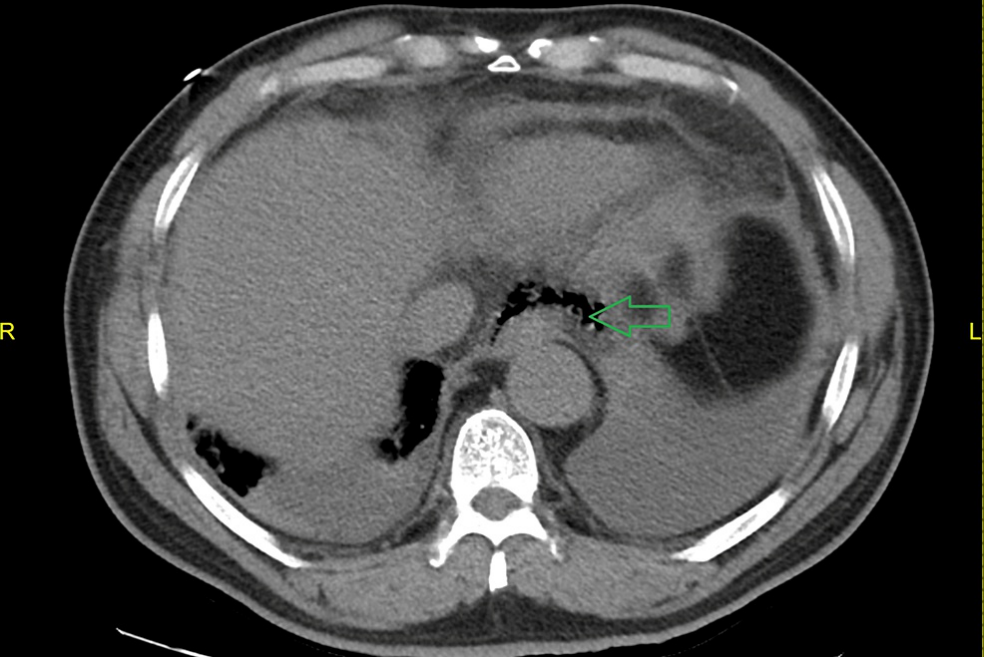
CT chest showing free gas surrounding the gastroesophageal junction in the lower aspect of the posterior mediastinum
